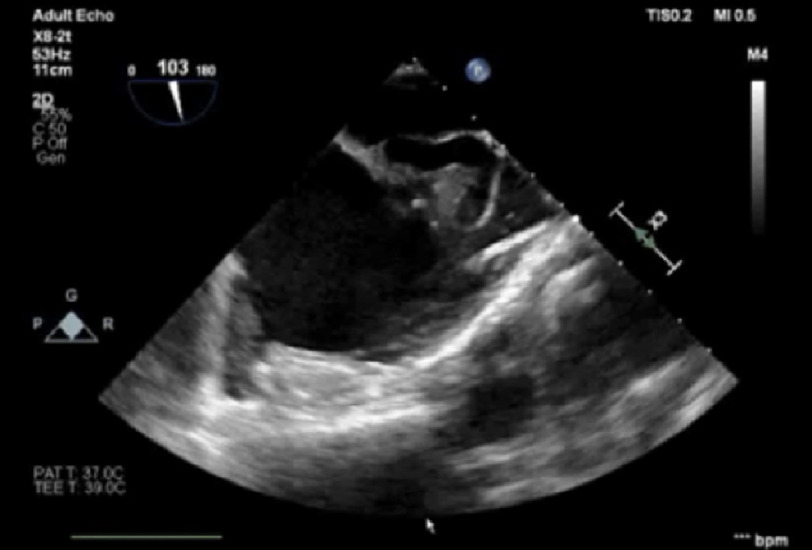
Transesophageal echocardiogram image demonstrating a coronary artery aneurysm of a branch from the right coronary artery.
